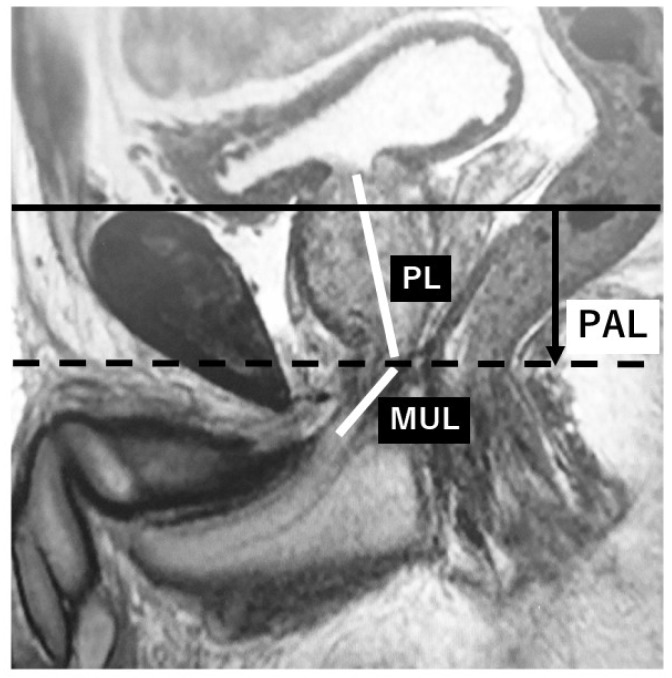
Preoperative parameters on sagittal MRI. (PL: Prostate length, MUL: Membranous urethral length, PAL: Pubic symphysis-prostate apex length, solid line: Extension line of the suprapubic ridge line, dotted line: Extension line of the prostate apical line.).
